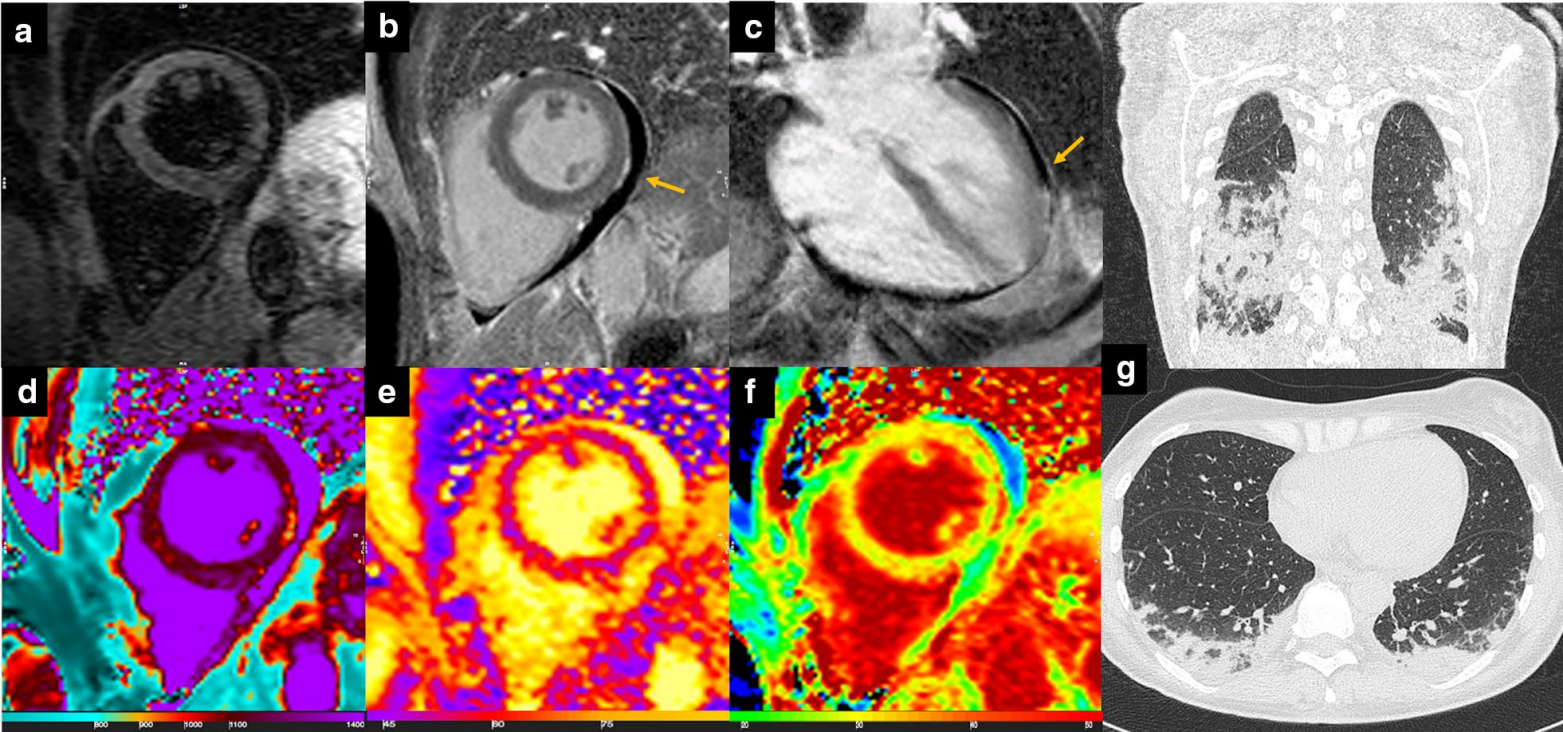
Pericarditis. 36-year-old in-hospital COVID-19 patient with chest discomfort and evidence of pericardial effusion at bedside Echo. a Evidence a slight hyperintensity of the pericardial layers, with no myocardial edema. LGE sequences confirm the presence on pericardial layers enhancement and moderate pericardial effusion (b, c orange arrows). Native T1 values were mildly increased (d) as well as T2 mapping (e) and ECV values (f). Coronal and axial chest CT revealed peripheral posterior areas of parenchymal consolidation (g). *Echo* echocardiography; *STIR* short tau inversion recovery; *LGE* late gadolinium enhancement; *ECV* extracellular volume; *CT* computed tomography
